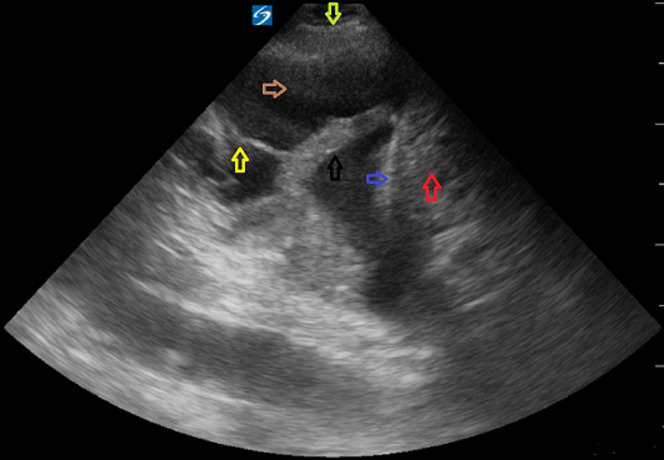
bedside ultrasound showing complex pleural effusion with cellularity (brown arrow), septations (yellow arrow) and atelectatic lung (black arrow). The diaphragm (indigo arrow), liver (red arrow), chest wall (lime arrow) are also visible
